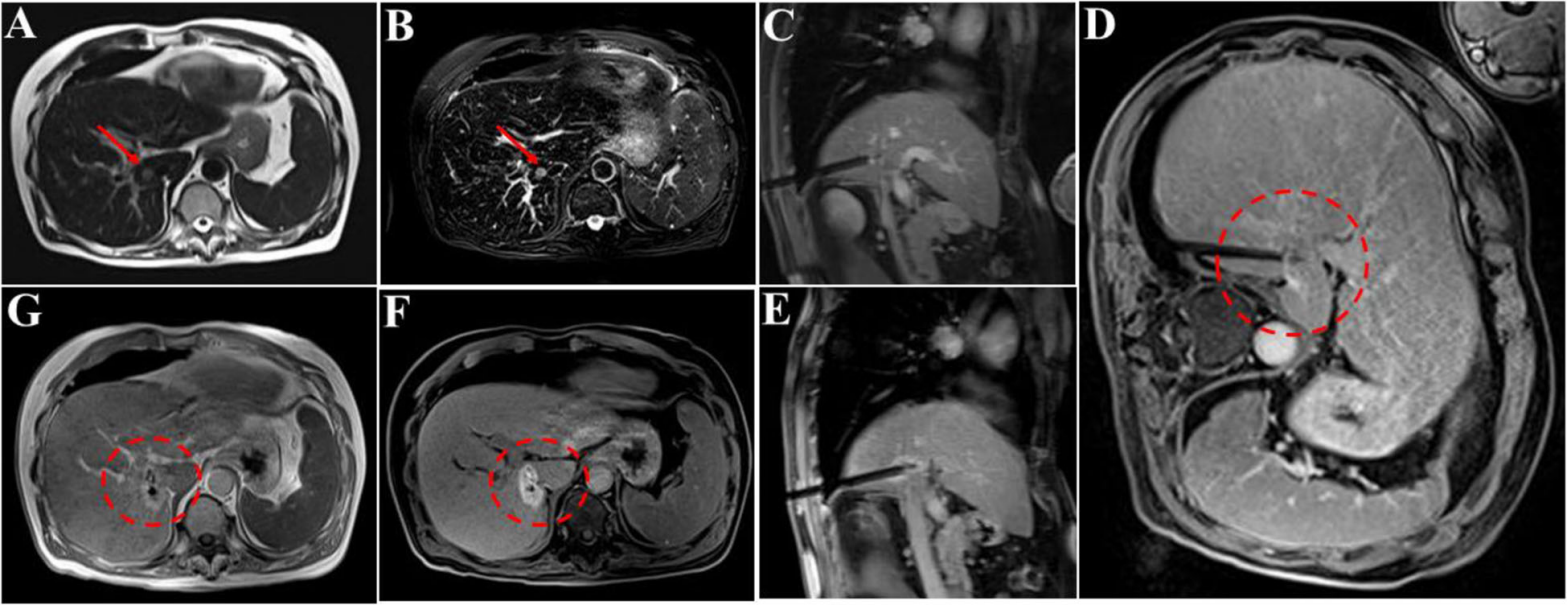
A patient completed MR-guided MWA treatment under general anesthesia. a-b The liver caudate lobe (red arrow) has a diameter of 17 mm and appears as hyper-intensity on T2-weighted transverse images before MWA. c-e The trajectory of the tilting puncture were adjusted gradually for the lesion target under the guidance of T1WI and the image shows precise insertion of the antenna into the target. f After 1 day of MWA, magnetic resonance reexamination found the treatment border covered the lesion completely as hyperintensity on T1WI (dashed circle). g Follow-up for 1 month, the lesion (red arrow) was completely ablated
